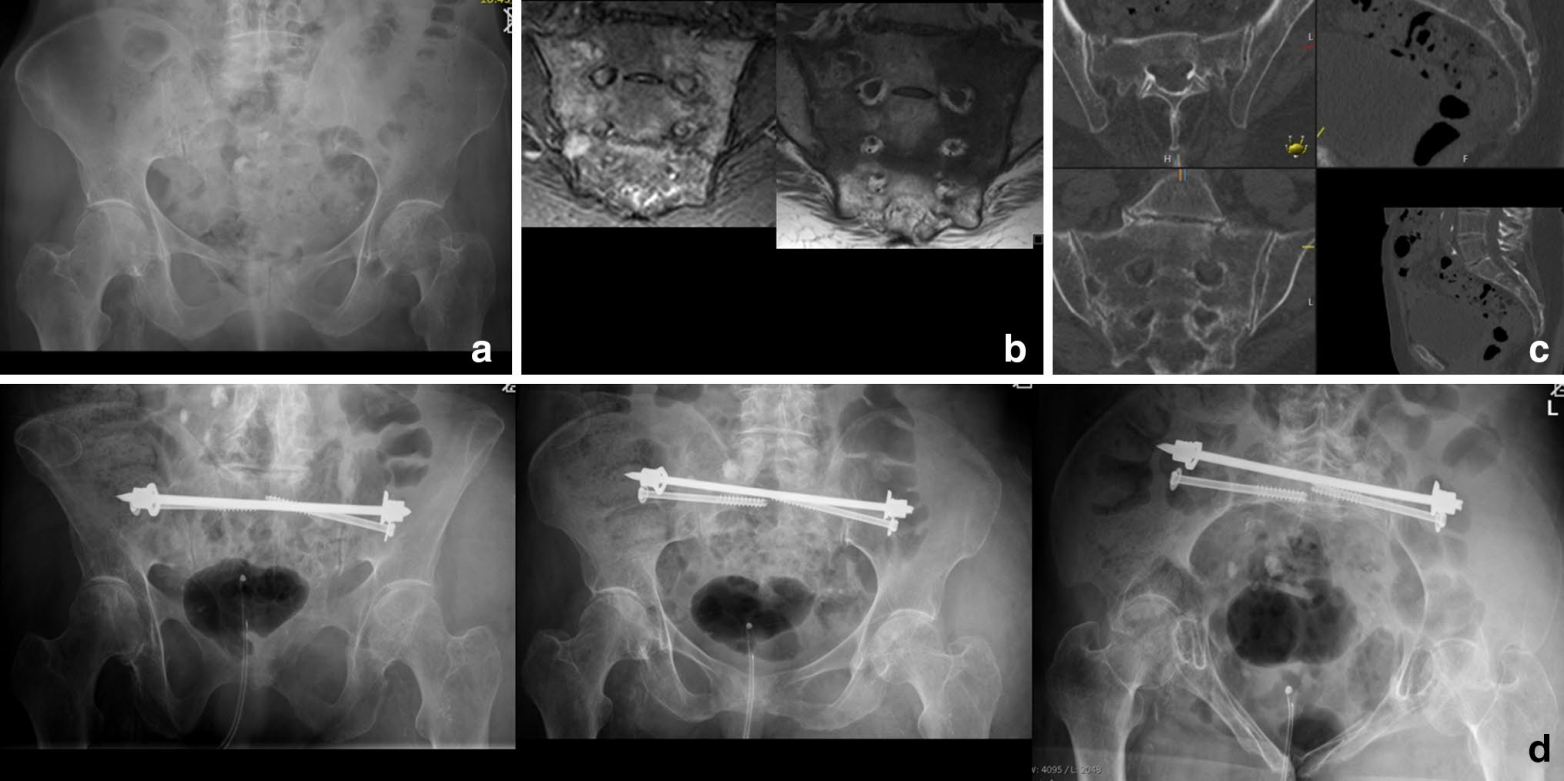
FFP type IIa. 84-year-old female with immobilizing lower back pain. Conventional radiograph did not show a bony lesion (a). Also with adequate pain medication mobilization was not possible. The MRI (T1 and STIR sequence in the coronal plane of the sacrum) showed bilateral bone bruise in the sacral ala with a transverse connection on level S2/S3 (b). A CT scan confirmed bilateral sacral involvement without fracture of the anterior pelvic ring (c). The patient was stabilized percutaneously with a trans-sacral bar and bilateral SI-screws (d)
